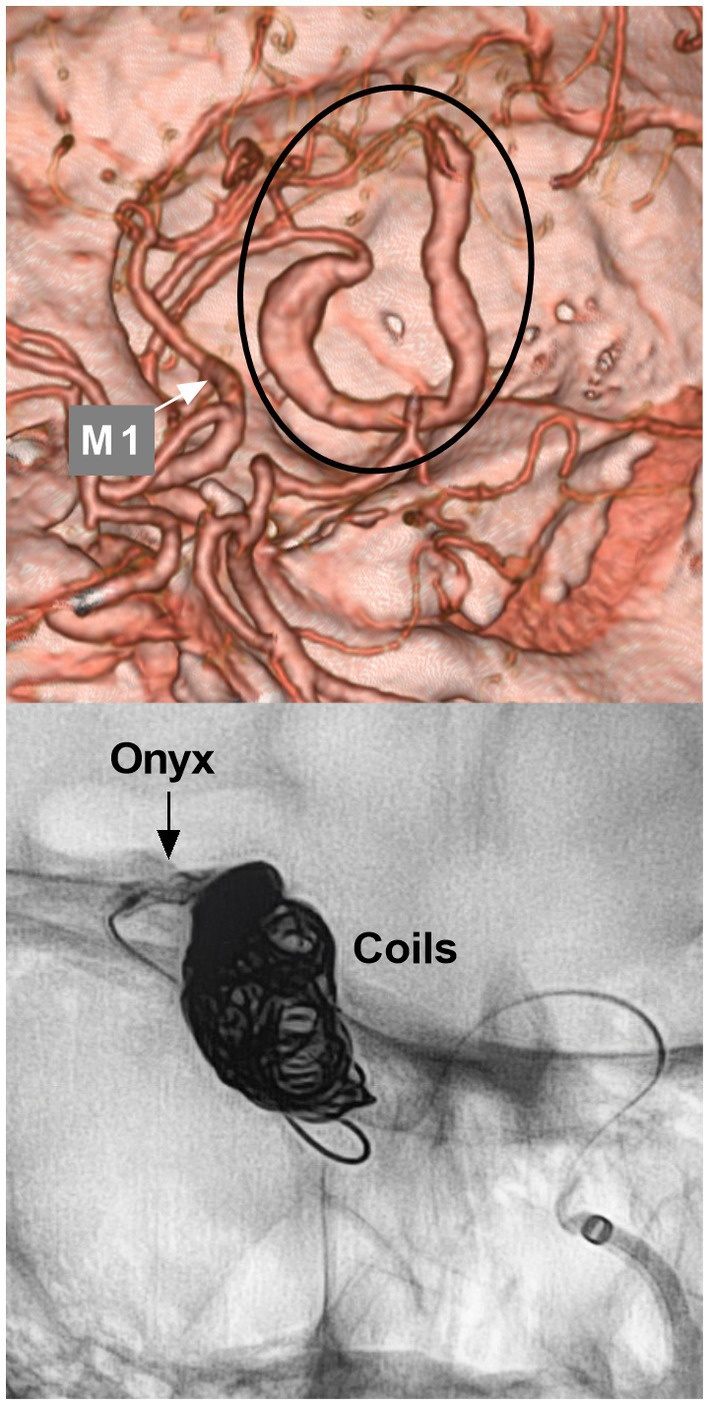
Parent artery occlusion in MCA aneurysm. Upper panel: CTA showing a serpentine aneurysm of the distal MCA; Lower panel: X-ray image showing parent artery occlusion of the aneurysm by coiling and Onyx casting. CTA, computed tomography angiography; MCA, middle cerebellar artery; M1, first segment of the MCA.
